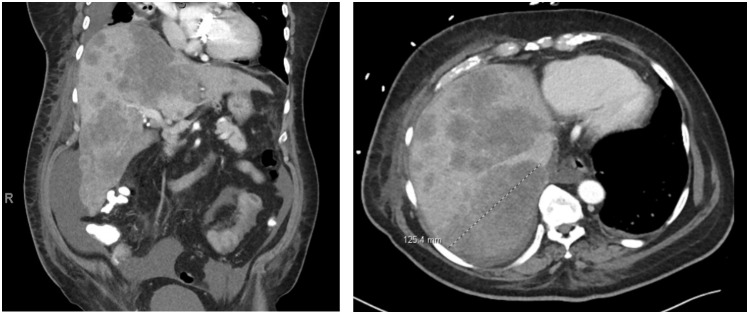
Coronal (left) and cross-sectional (right) view of CT chest/abdomen/pelvis
